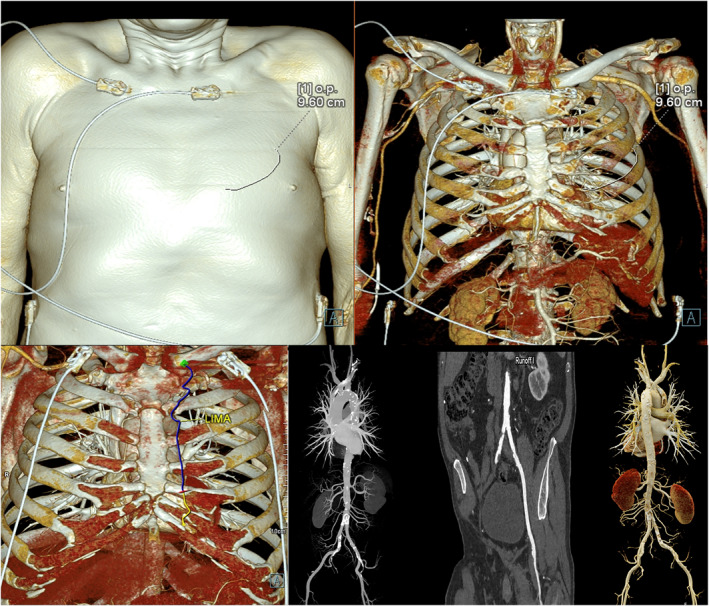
Computed tomography images of the thorax anatomy, LIMA topographic anatomy and peripheral arterial system and the aorta to guide pre‐operative planning.
